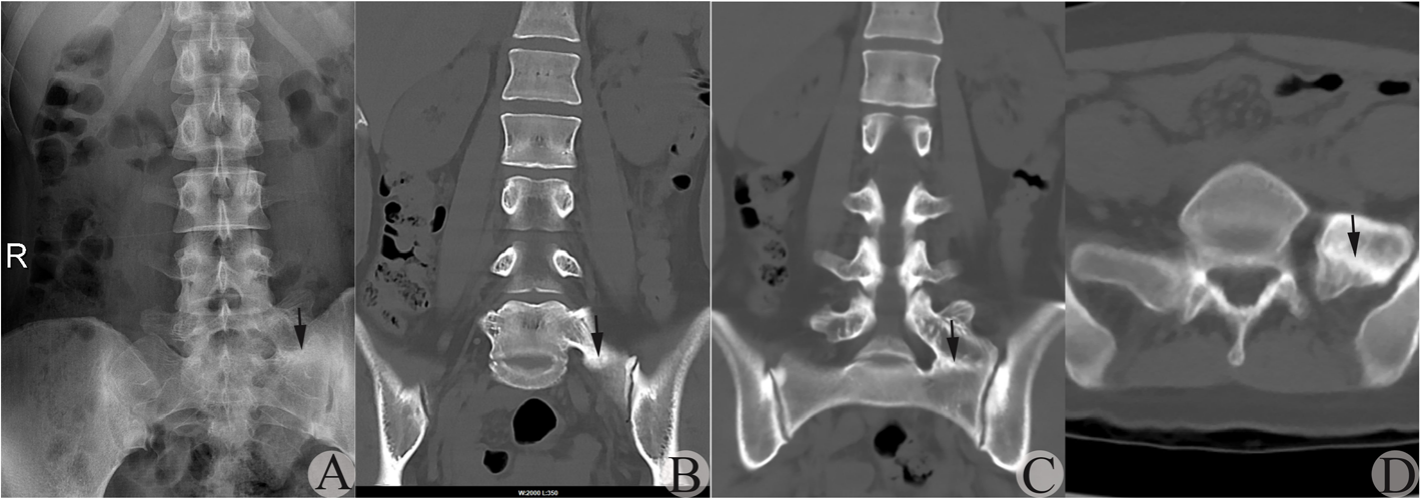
A suspected type IIa MA-LSTV judged by AP-LPR was verified to be type IIIa by CT-CRI. a AP-LPR image showing the suspected JLS on the left side. Arrow indicates the suspected JLS. b CT-CRI through vertebral body planes showing the suspected JLS to be RSB located at the bony fusion region. Arrow indicates the BUS with RSB. c CT-CRI through vertebral arch showing the suspected JLS to be RSB located at the bony fusion region; no JLS was detected. Arrow indicates the BUS with RSB. d Transverse CT image showing the BUS with RSB on the left side. Arrow indicates the BUS with RSB on the left side
